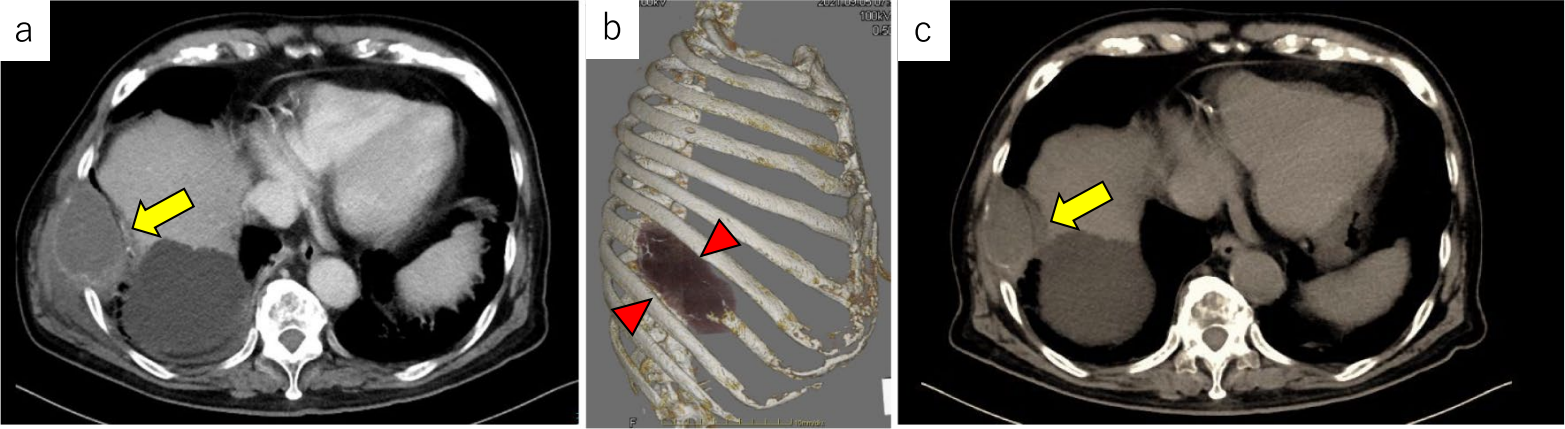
CT scan images of the chest wall tumor. a Axial CT image at the first visit. b 3D-reconstructed CT image of the tumor. c CT scan image of the tumor with spontaneously regression after 1 month
